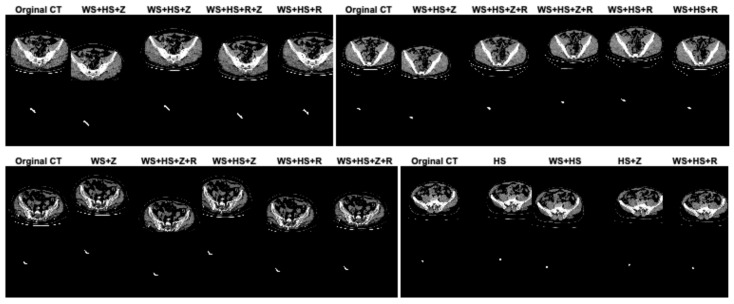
The effect of the data augmentation procedures on some CT scans and GT masks in the dataset.
